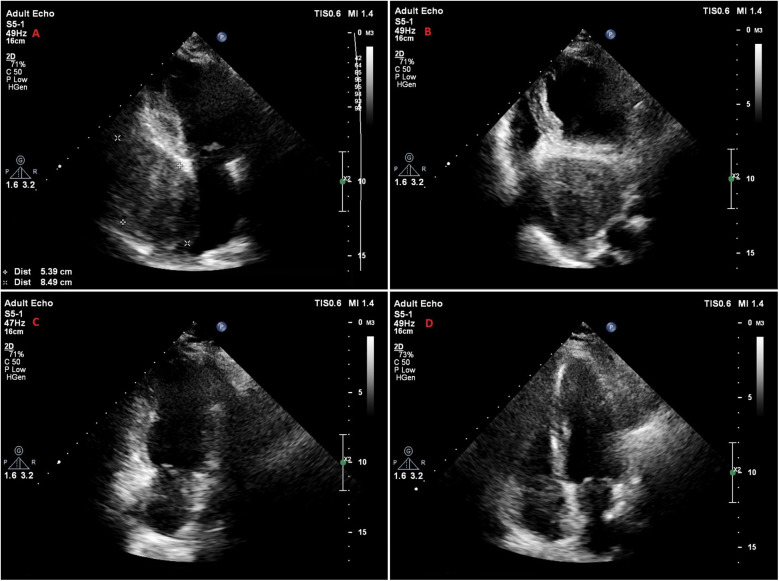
Transthoracic echocardiography images of the patient. (A,B) Apical 2-chamber (A) and apical 4-chamber (B) views of a hypoechoic mass located posterior to the left atrium and left ventricle, and compressing the left atrium. (C,D) Apical 2-chamber (C) and apical 4-chamber (D) views of the patient at one year follow-up, showing that the mass was successfully removed.
